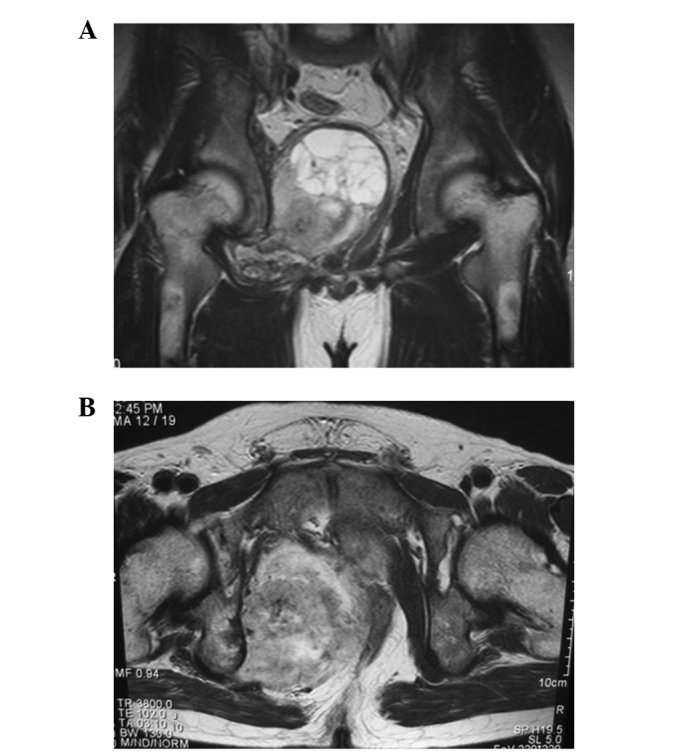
Case two: (A) Coronal and (B) axial T1-weighted gadolinium-enhanced magnetic resonance imaging (MRI) revealing a heterogeneous intensity mass, with partial cystic change, compressing the rectum and bladder.
